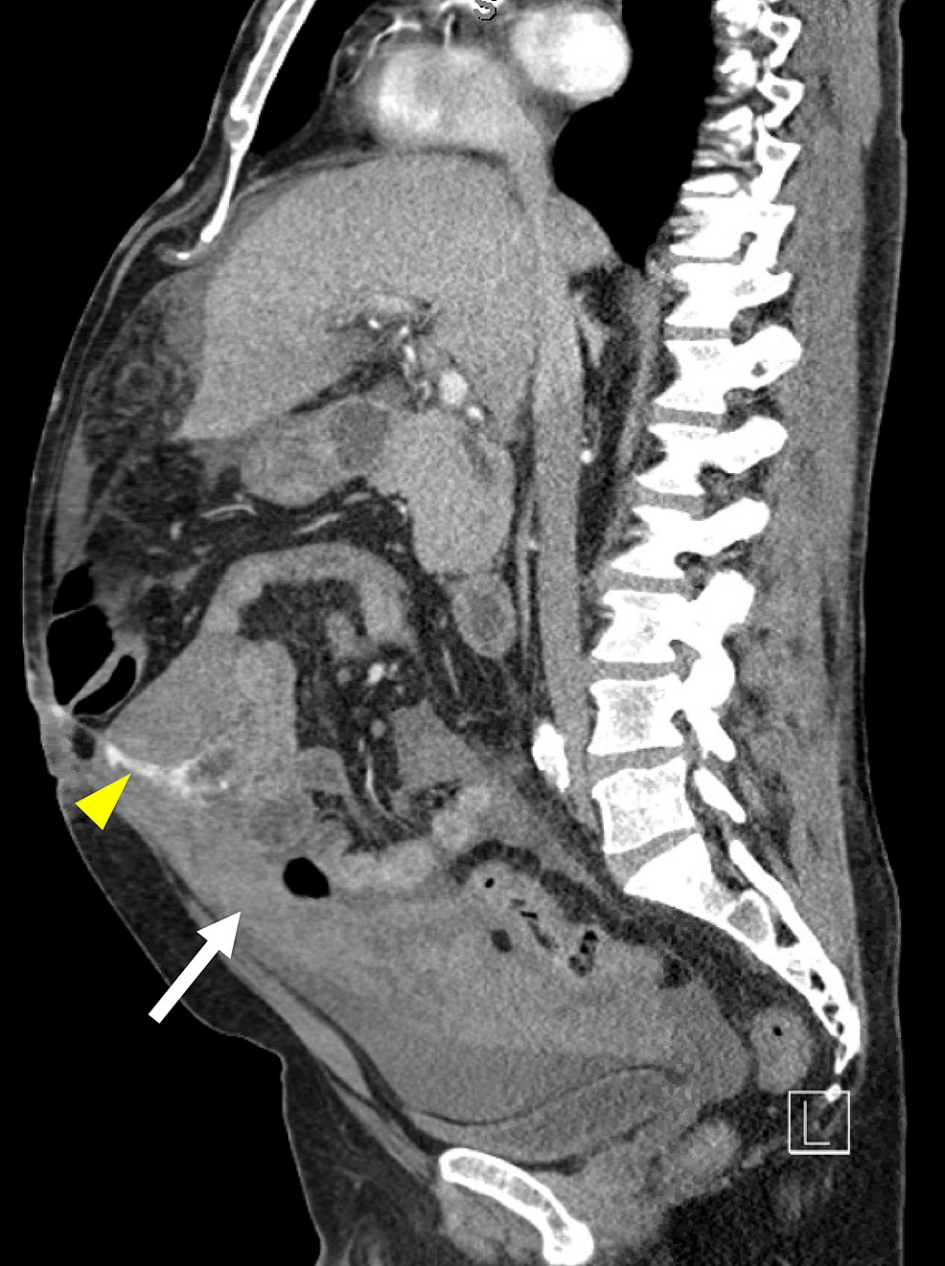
CT with angiography showing hemoperitoneum (white arrow) with possible source of hemorrhage from portosystemic collateral (yellow arrow) in the region of greater omentum and small bowel wall; sagittal view.
